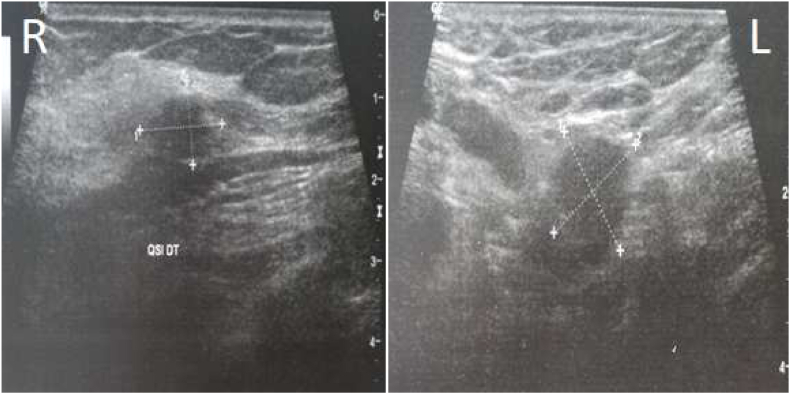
Mammary ultrasound: A right and left breast lump which are classified respectively BI-RADS V and BI-RADS IV.
